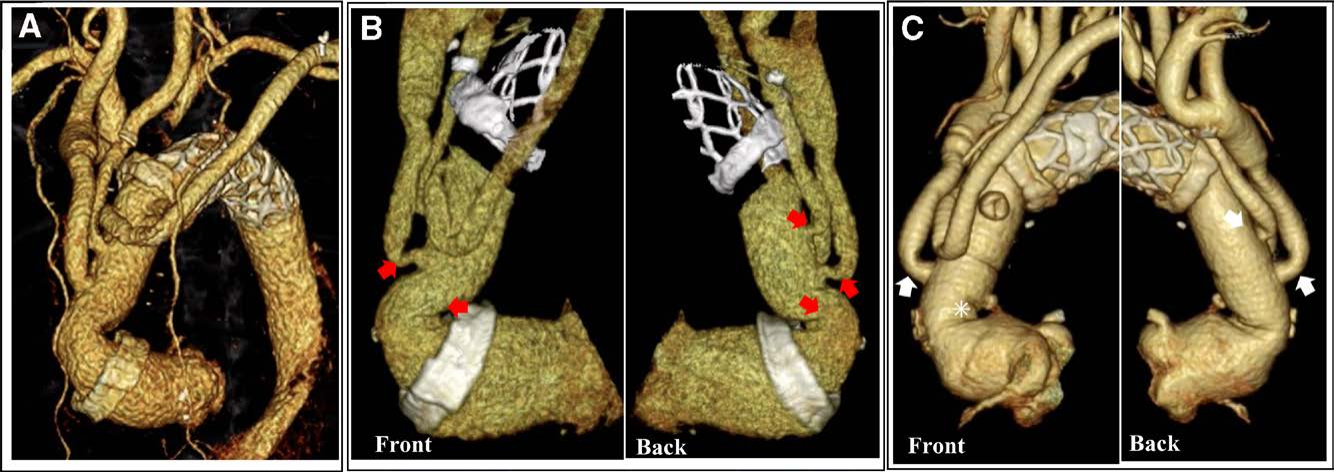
Pre- and postoperative CT images. (A) A volume-rendering image captured after the first surgery is presented. (B) An image taken before the revision surgery is presented. The red arrows show suspicious lesions of the kinked graft. (C) An image after the revision surgery is presented. The white asterisk shows the adequate length of the ascending portion of the graft. The white arrows show the result of the adequate angle of the first branch, but the second branch was unchanged. CT = computed tomography.
